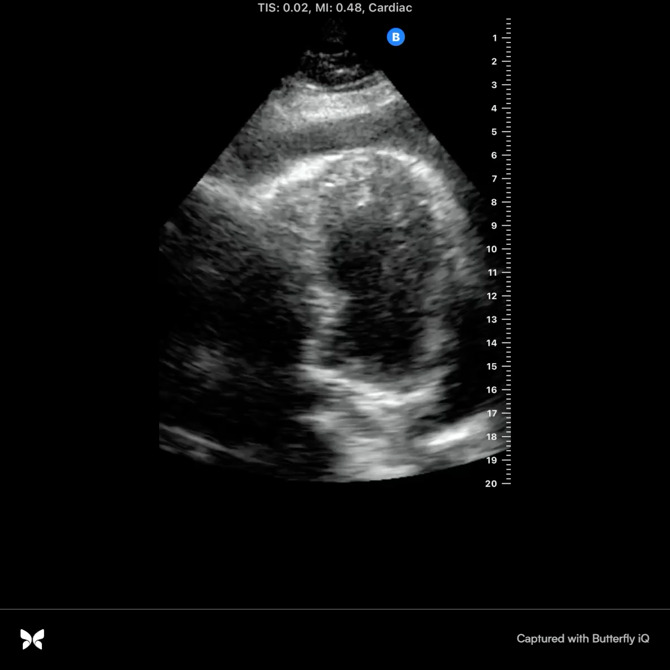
Apical four chamber view by point of care cardiac ultrasonography revealing moderate to large pericardial effusion. This view is foreshortened and unable to completely visualize the atria therefore limiting interpretation.
